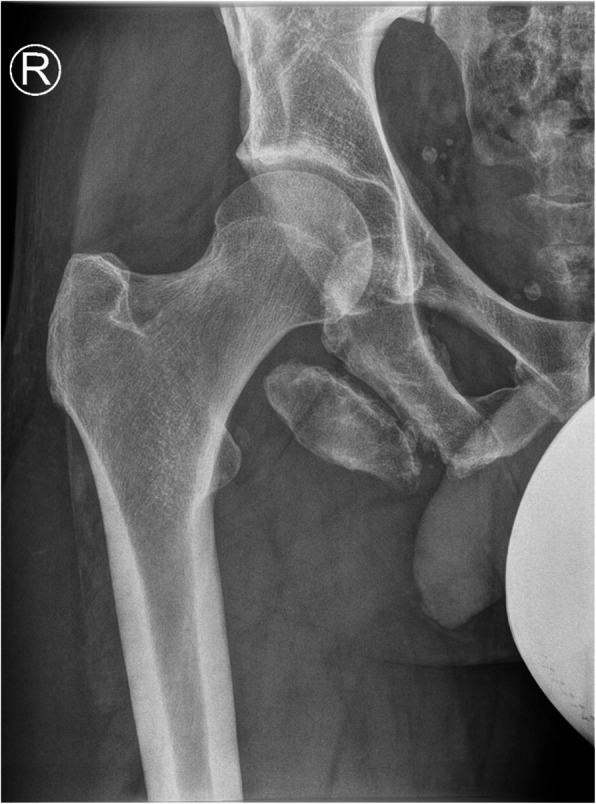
Preoperative radiograph showed right ischial tuberosity apophyseal fracture with a large displaced fragment
